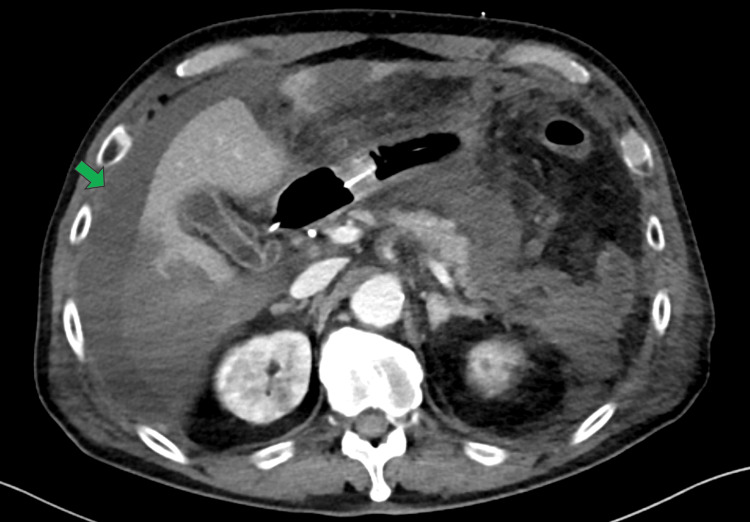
Abdominal CT on admission to our hospital showing new-onset ascites (green arrow) without evidence of a pancreatic pseudocyst CT: computed tomography
